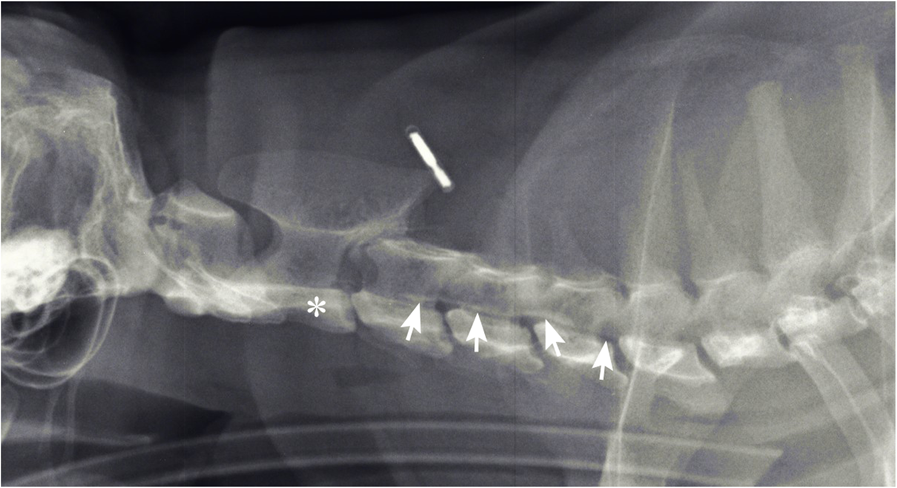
Lateral radiograph of the cervical vertebral column. Thin radiopaque line (pointer arrows) appears ventrally in the vertebral canal dorsal to vertebral bodies C3-5. The asterisk corresponds to the second cervical vertebra.
